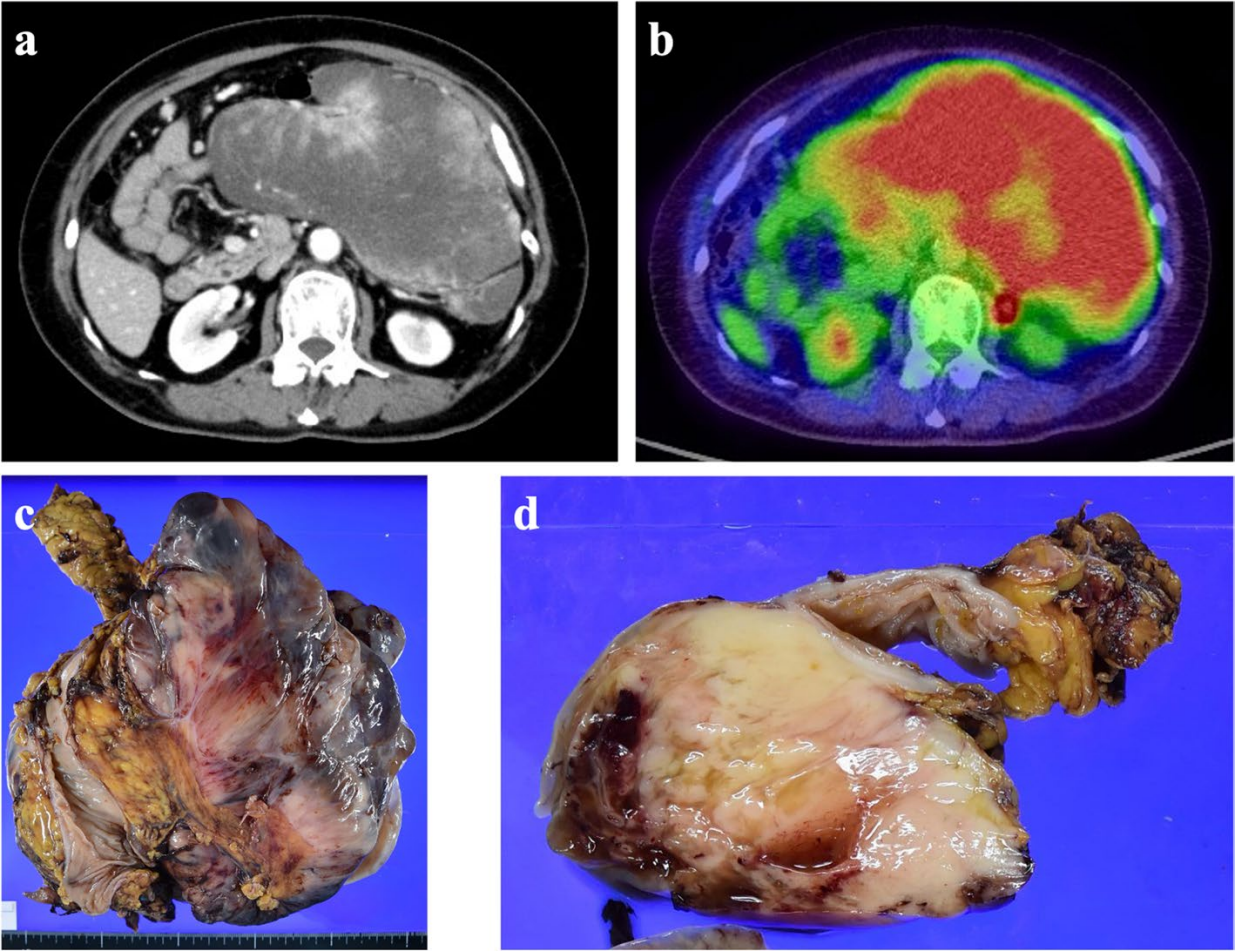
a Contrast-enhanced CT findings revealed a large submucosal mass approximately 180 mm in length, contiguous with the transverse to descending colon. Heterogeneous contrast images were visible within the mass. b PET-CT demonstrated FDG accumulation (SUVmax: 9.42) within the mass, with no evidence of obvious abnormal accumulation elsewhere. c The resected intra-abdominal tumor was 260 × 250 × 120 mm in size and weighed approximately 3.5 kg. d The cut surface of the mass was grayish-white, with a circumscribed surface, and an extramural growth from the transverse colon. Mucinous degeneration was observed in certain areas along with a certain degree of bleeding
